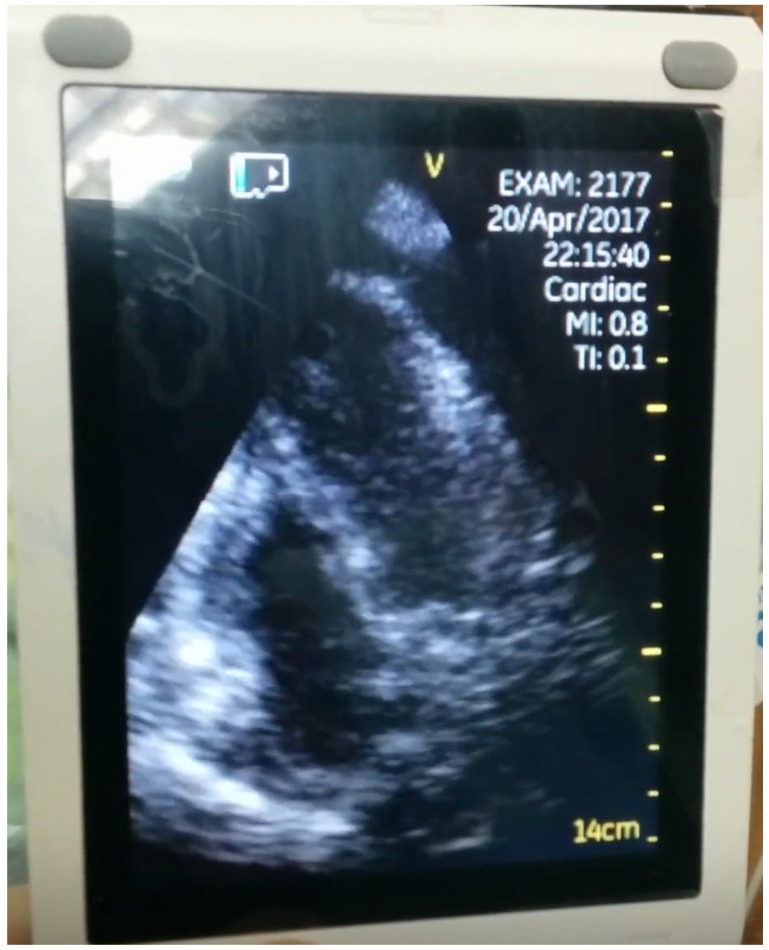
Point-of-care ultrasound of a patient with clinical signs of tamponade. The picture is a screenshot from a mobile phone, as at this point of care ultrasound devices in our institution do not produce DICOM images but rather allow real-time imaging only.
